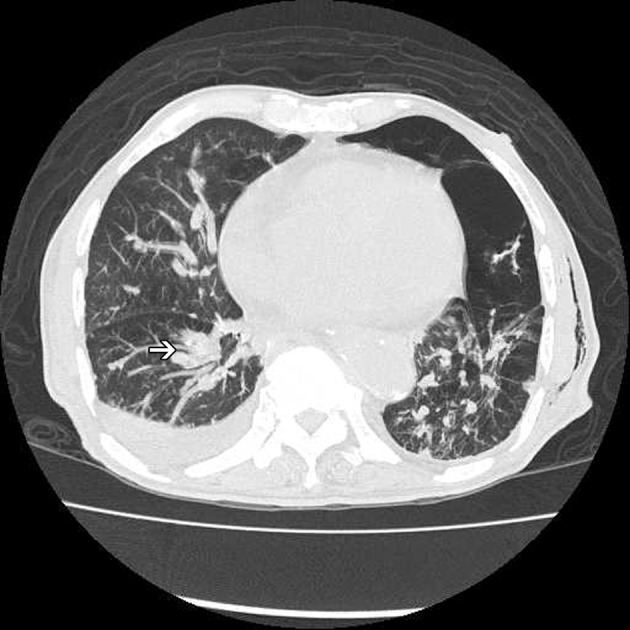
High resolution computed tomography (HRCT) of the chest showing hilar lymphadenopathy (arrow) and diffuse reticular nodular lesions over bilateral lung, with left residule pneumothorax and subcutaneous emphysema over left chest wall.
